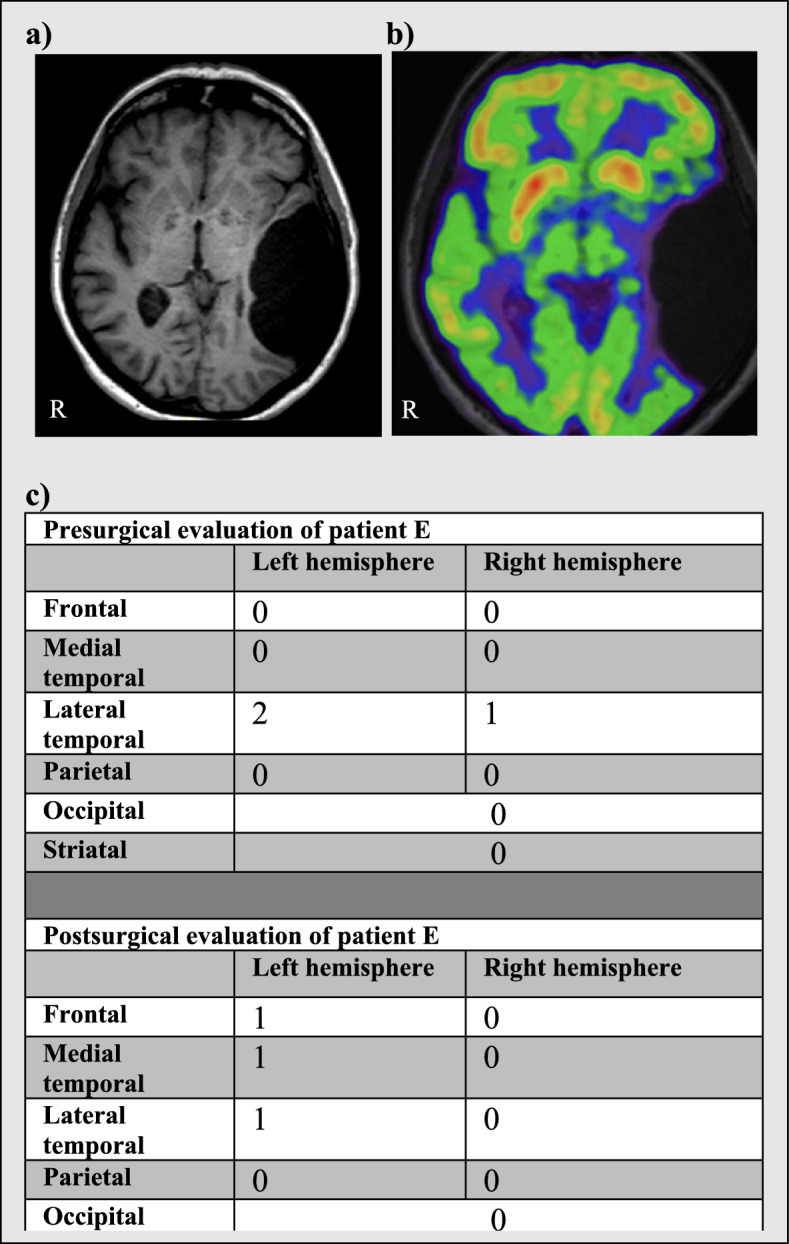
Patient E. Patient E was an 85-year-old female with complaints of impaired memory, word retrieval, reading and writing skills. New MR images revealed that the previously detected arachnoid cyst in the left temporal lobe had expanded from a volume of 69 cm^3^ to a volume of 96 cm.^3^ over the course of 24 months and that a midline shift of 5 mm had developed. It was now classified as a Galassi type 3 arachnoid cyst. An FDG scan showed focally reduced uptake in the right cerebellar hemisphere. Neuropsychological examination indicated impairment of the bilateral temporal areas, most severely in the left hemisphere. The patient had surgery which was followed by disappearance of the mass effect and an extensive reduction of the cyst’s volume on MR. The patient reported subjective improvements of complaints but the neuropsychological evaluation 11 days after surgery did not show clear objective improvement. FDG scanning was not repeated. Dementia was not diagnosed. a MR. b FDG-PET. c Neuropsychological scores (see 2.2 Neuropsychological profiles for explanation)
